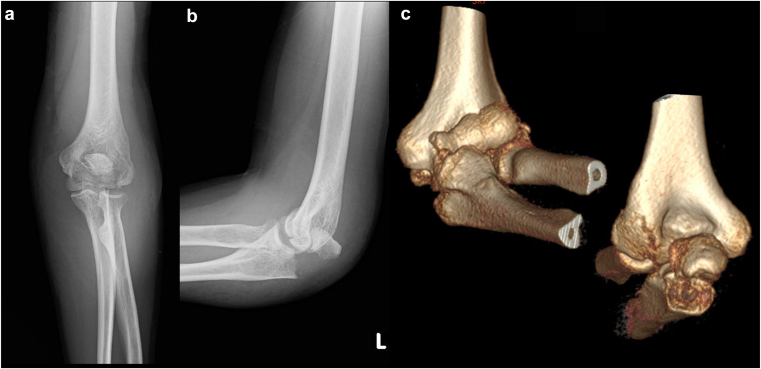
Preoperative plain radiographs and CT images. (a) Anteroposterior radiograph, (b) lateral radiograph, (c) 3D CT reconstruction. *CT*, computed tomography; *3D*, three-dimensional.
